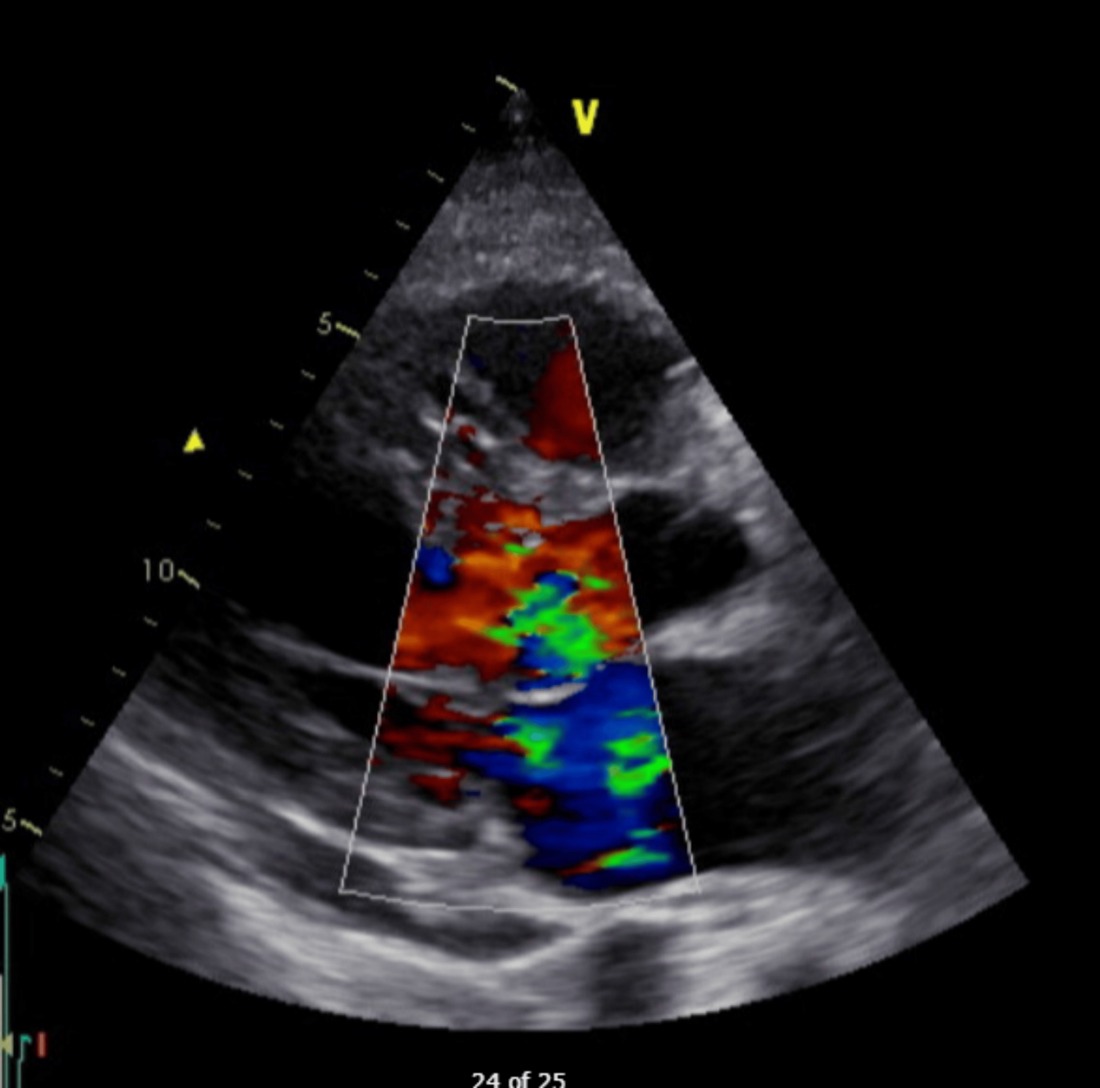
PLAX showing grade II mitral regurgitation. PLAX: Parasternal long axis.
